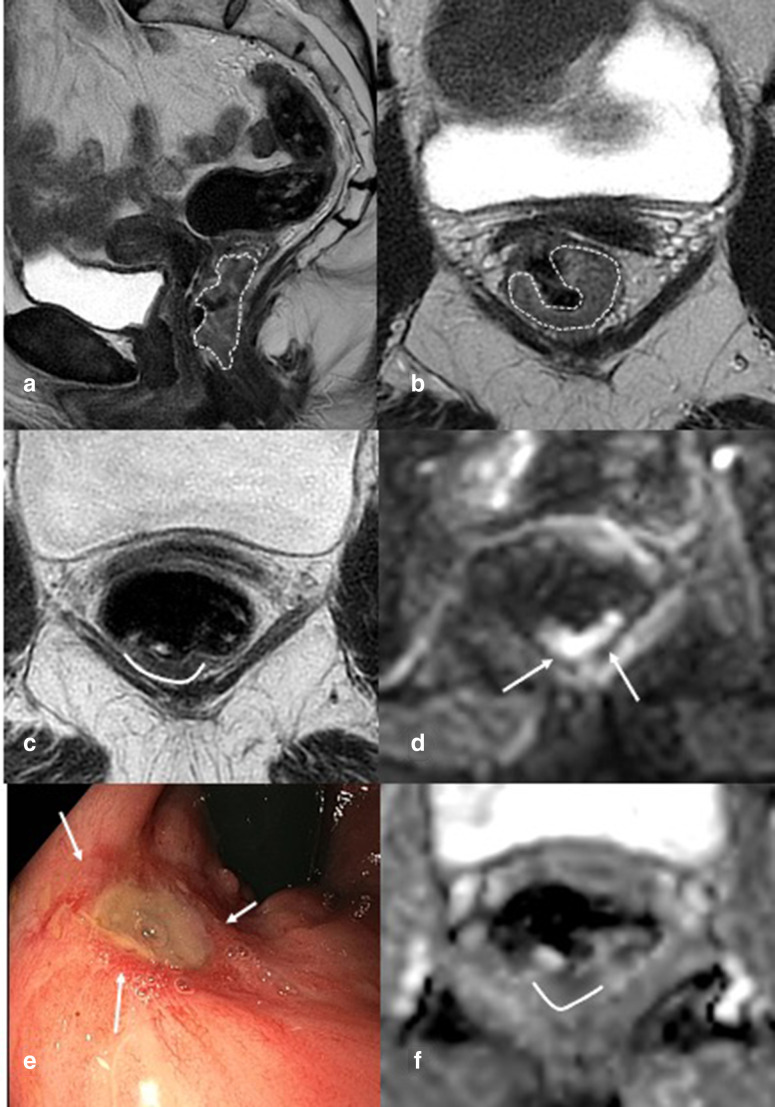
Small remnant. In (a/b), sagittal- and axial-weighted images of distal semi-circular tumor (outlined with dotted line), invading the anal canal. (c) Shows an axial T2-weighted image 12 weeks after chemoradiation. A small quite homogeneous wall thickening is visible with intermediate signal (outlined dorsally with a white line) and diffusion restriction (white arrows in d and line on ADC map in f). On endoscopy (e), an ulcer (deeper than the ulcer in Figure 3) with irregular edges is found (white arrows) and with DRE an irregular border is palpable. DRE, digital rectal exam.
